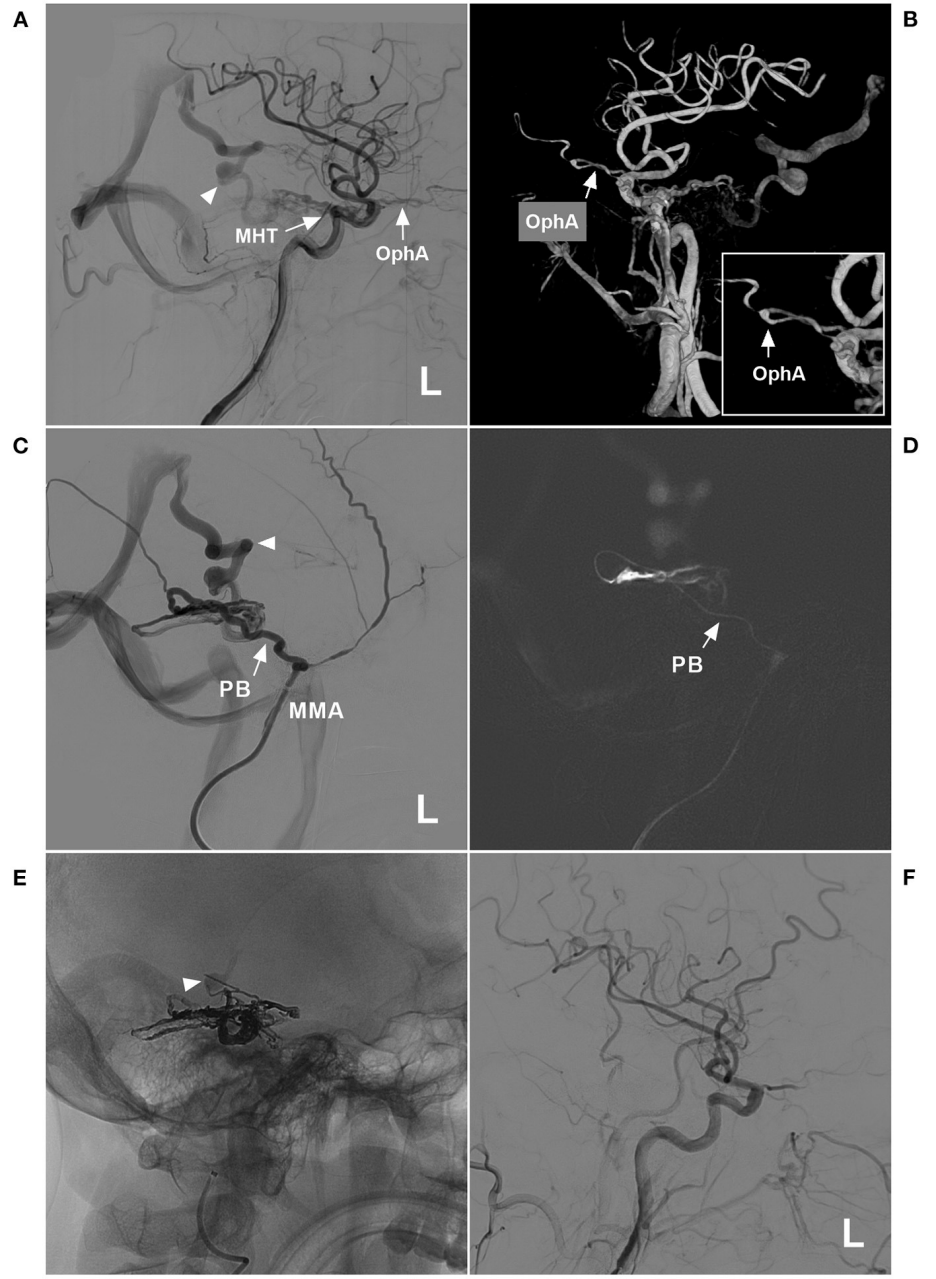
Successful type IIIb EVT for a DAVF of the tentorium. (A) Lateral view DSA of the left carotid artery showed a DAVF supplied by the MHT and OphA (arrows) draining to the vein of Galen (triangle). (B) Three-dimensional DSA showed that the recurrent meningeal branch of the OphA supplied the DAVF (arrow). The inset in the lower right shows the magnified local OphA (arrow). (C) Left MMA angiography showed that the PB of the MMA supplied the DAVF. The triangle indicates deep vein drainage. (D) A roadmap image shows that the microcatheter passed through the PB (arrow) and into the fistula. (E) X-ray film showing the casting Onyx. Onyx penetrated the fistula and sealed the draining vein (triangle). (F) The left carotid artery showed that the EVT was complete. DAVF, dural arteriovenous fistula; DSA, digital subtraction angiography; EVT, endovascular treatment; L, left; MHT, meningohypophyseal trunk; MMA, middle meningeal artery; OphA, ophthalmic artery; PB, petrous branch.
